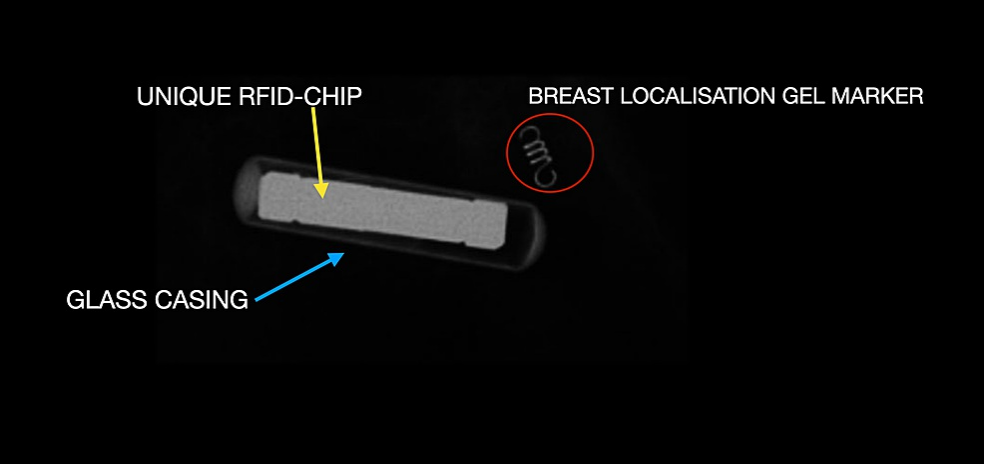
Structure of an RFID The magnified radiograph of an RFID device and HydroMark® clip (Mammotone, Cincinnati, Ohio). The chip (yellow arrow) can be seen encased in a glass casing (blue arrow). The total unit measures 9 mm. The commonly used Gel Mark clip can be seen for comparison of size and appearance (red circle). RFID: radio-frequency identifier device
